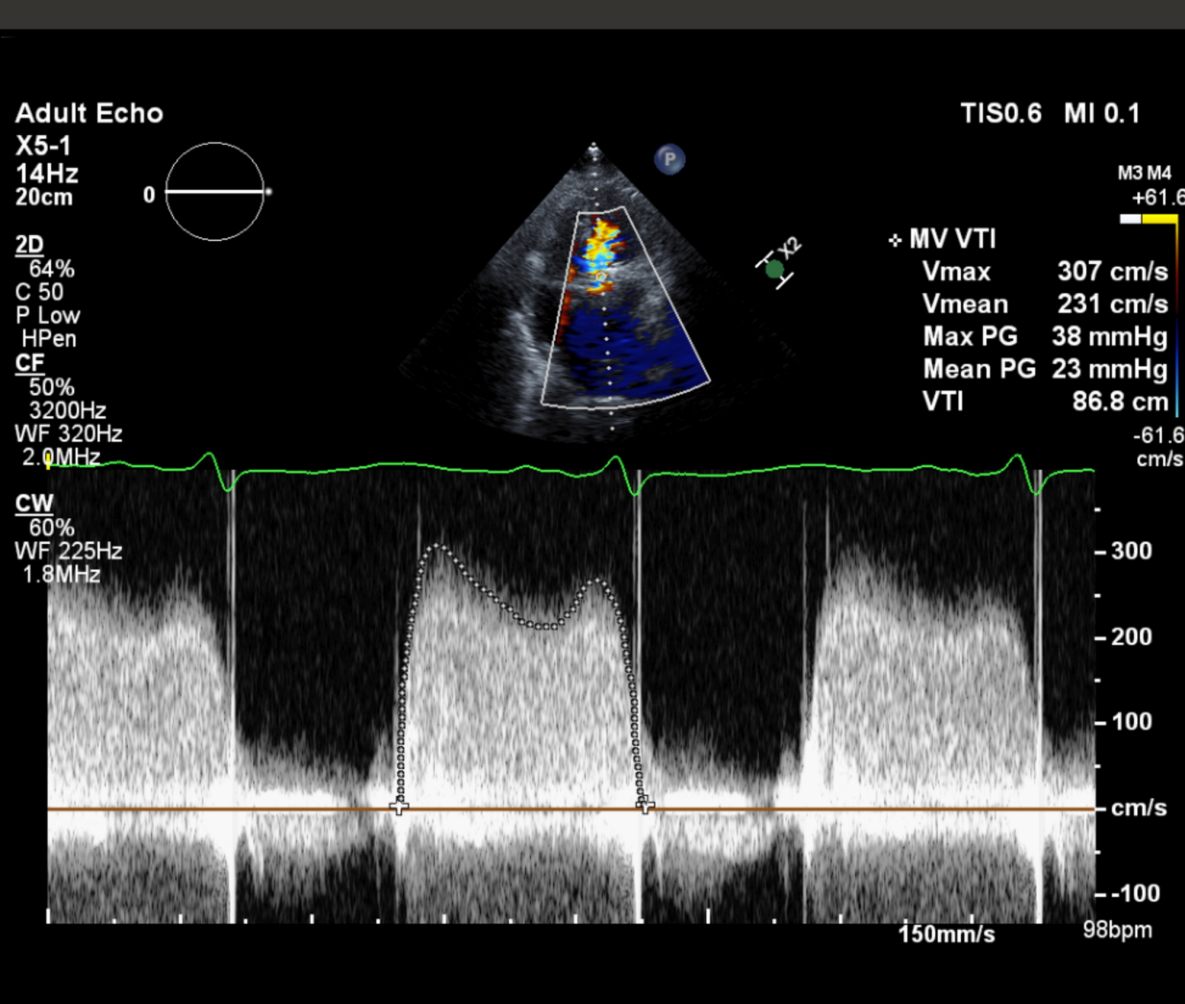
Transthoracic echocardiography doppler measurements showing increased mean pressure gradient across mechanical mitral valve
